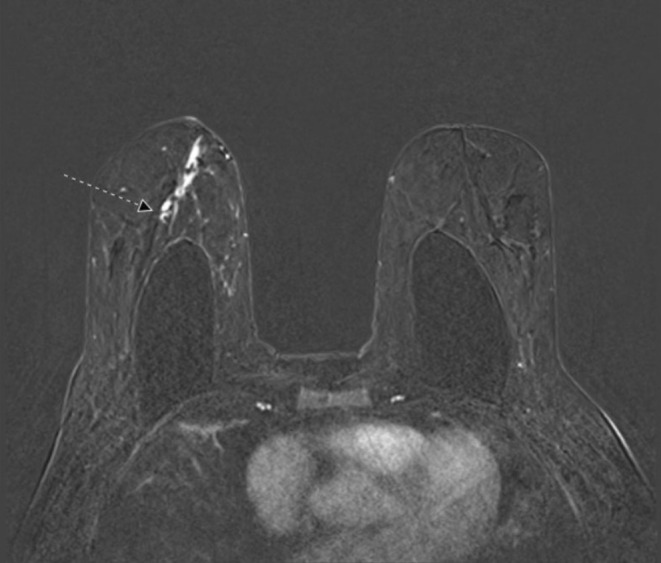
Breast MRI, bilateral, subtracted view, showing the 3.3 cm area of clumped and linear nonmass enhancement in the right medial, central, and retroareolar region, 0.9 cm from the base of the nipple and approaching the anterior aspect of the intact retropectoral implant (arrow).
